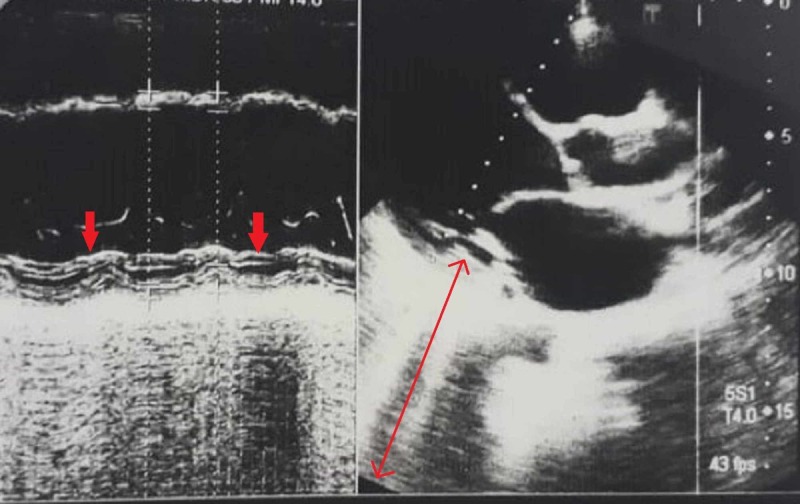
TTE showing concentric LV hypertrophy TTE: transthoracic echocardiogram, LV: left ventricle
